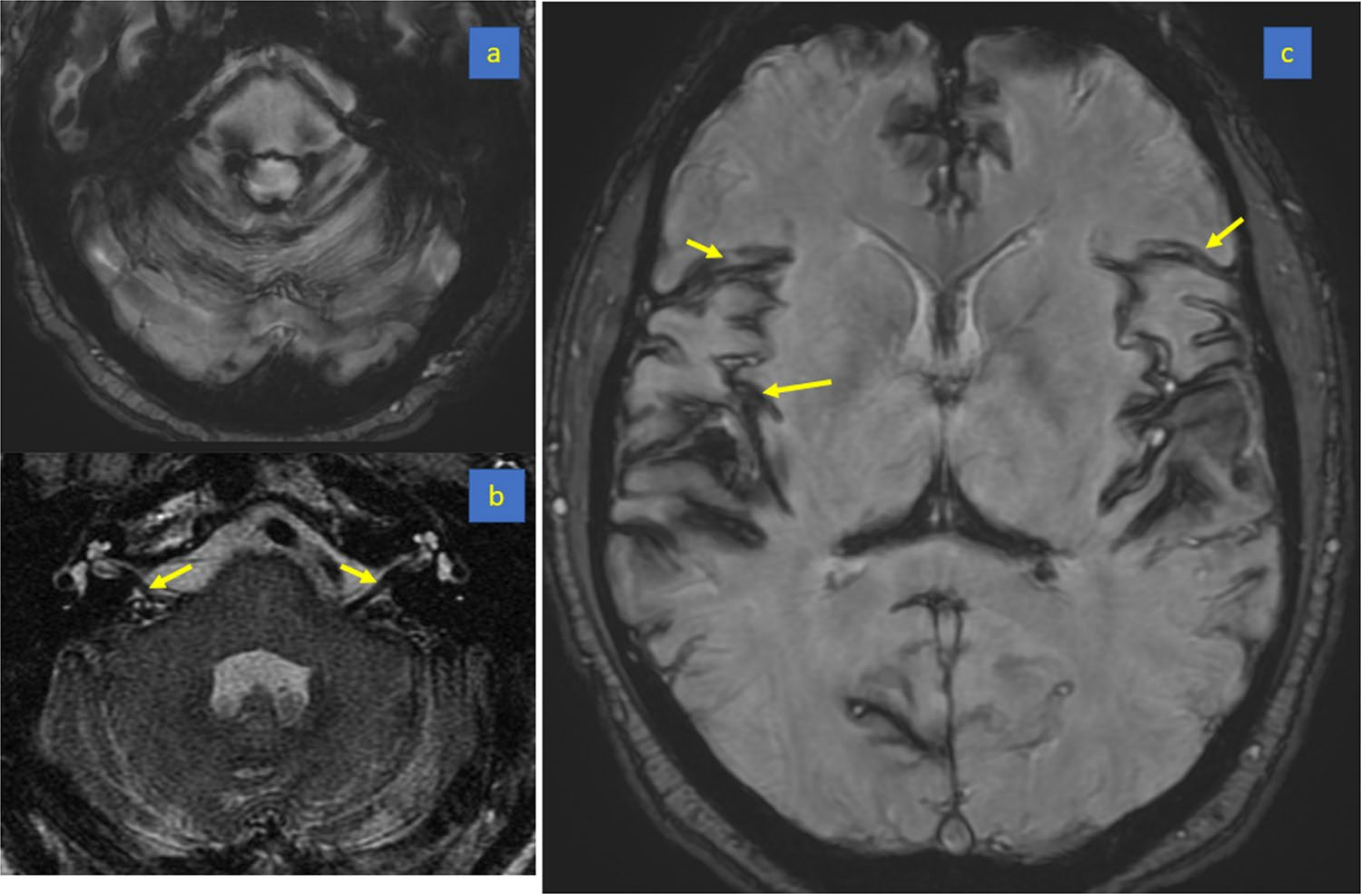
Patient (4). Susceptibility weighted brain MRI showing extensive leptomeningeal low signal intensity around the cerebellar folia (a), the basis pontis and vestibulocochlear nerves (b) and Sylvian fissures (c) due to hemosiderin deposition
